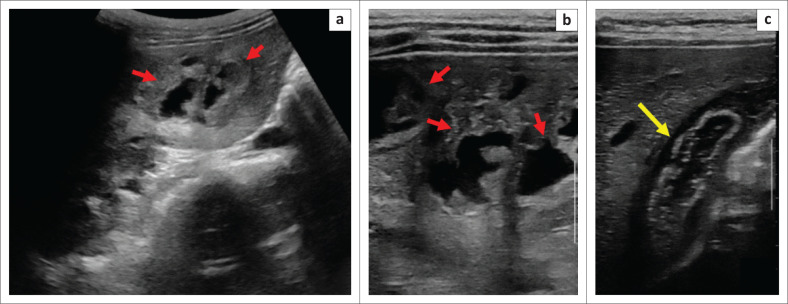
Ultrasonography of the liver. Image (a and b) showing multiple clustered hypo and anechoic complex cystic lesions (red arrows) with a mildly hyperechoic rim. Image (c) showing an oedematous gall bladder wall (yellow arrow).
